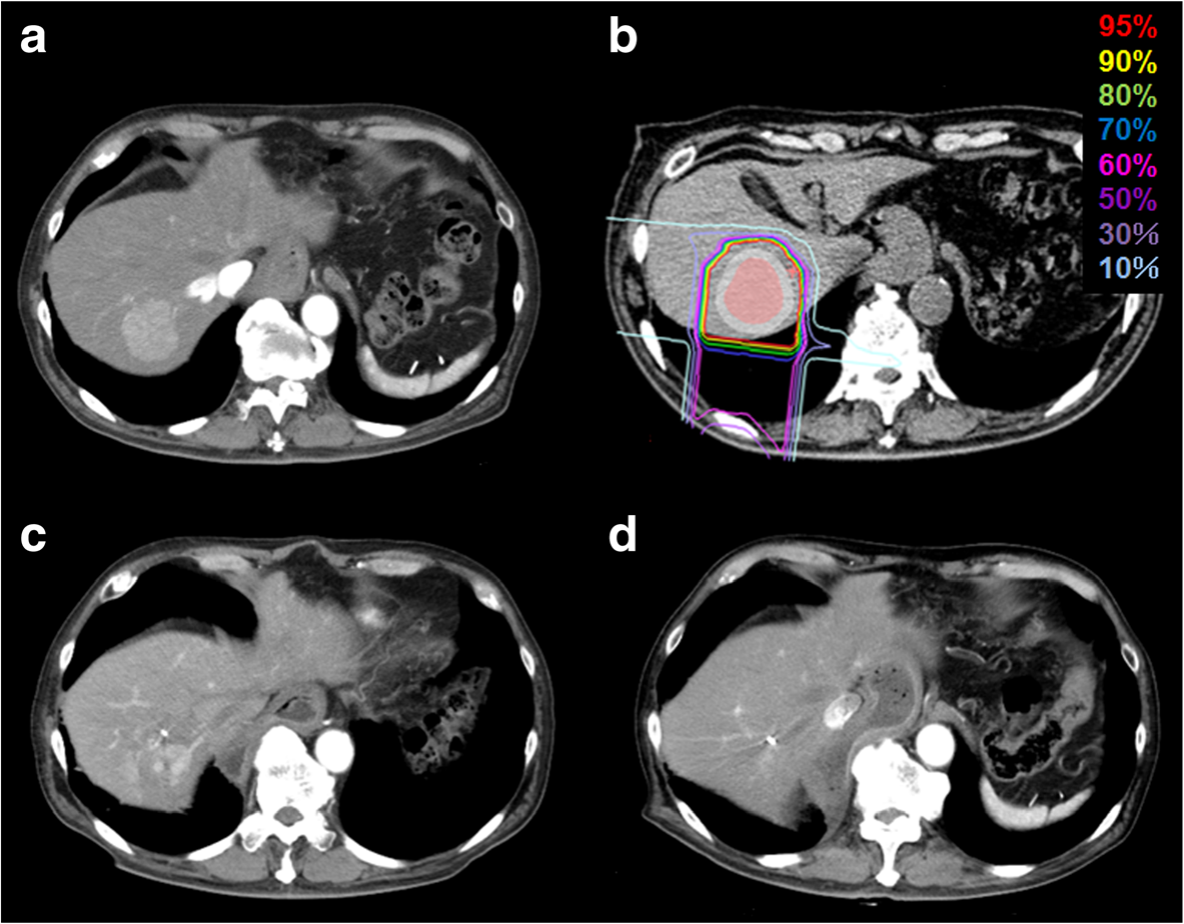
An 80-year-old male with HCC treated with C-ion RT. a CT before treatment. b Dose distribution on axial CT images. The area within the red outline is GTV and the area within the pink outline is CTV. c CT 3 months after treatment. d CT 18 months after treatment. There was a gastric tube reconstruction of esophageal cancer after surgery to the right of the vertebrae
